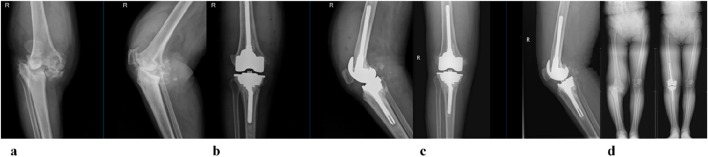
A 65-year-old female patient with AORI Type III post-traumatic knee arthritis (PTKA) underwent 3D-printed integrated trabecular prosthesis (ITP) knee reconstruction. (a) Preoperative X-ray; (b) X-ray at 1 week post-surgery; (c) X-ray at the final follow-up; (d) Comparison of preoperative and postoperative lower extremity alignment.
